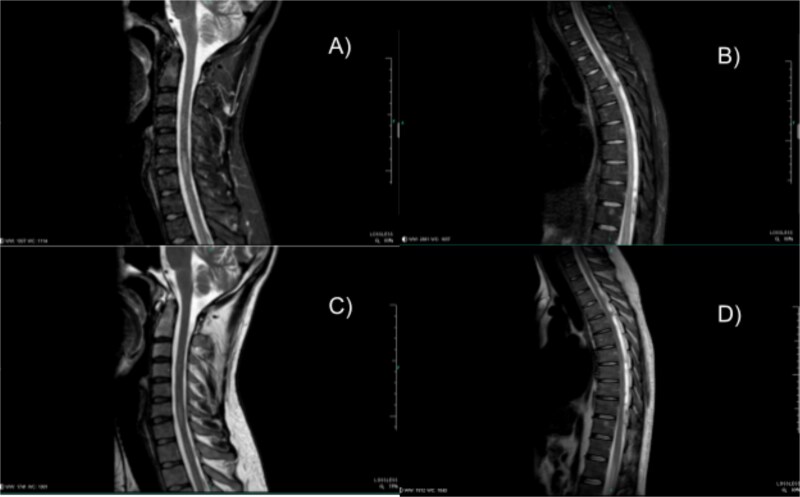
Spine MRI with hyperintense signal on T2 and STIR in the anterior aspect of the spinal cord at C6-C7, without gadolinium enhancement, and linear hyperintense signal in the thoracic spine, extending from T8-T10. (A) Sagittal STIR cervical. (B) Sagittal STIR thoracic. (C) Sagittal T2 cervical. (D) Sagittal T2 thoracic.
